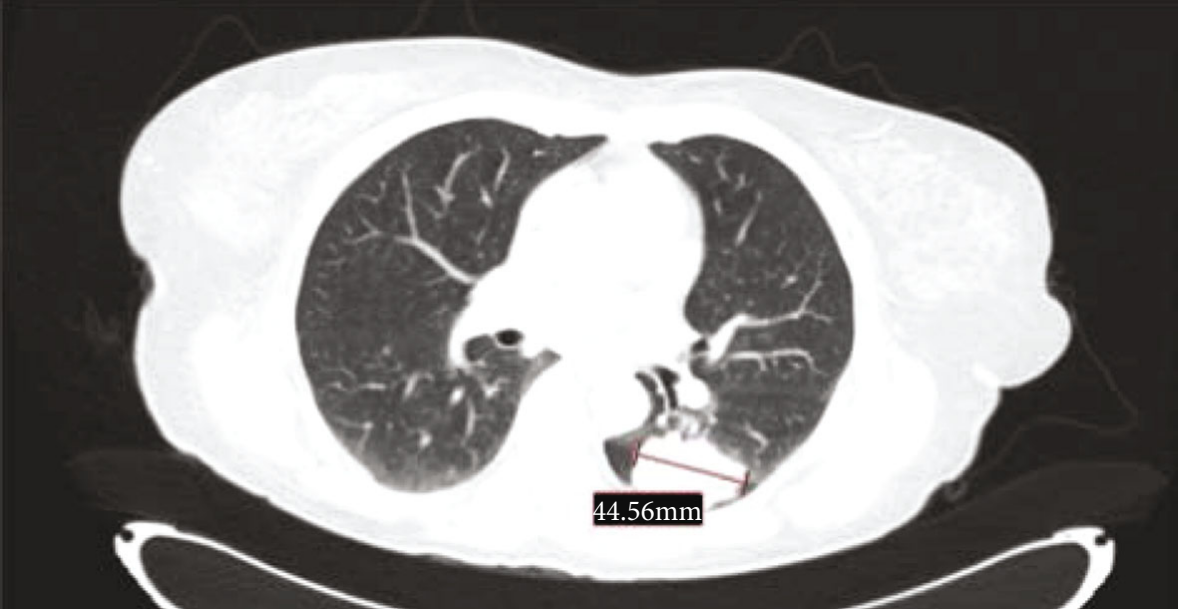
Lung mass on thoracic CT scan at diagnosis.
